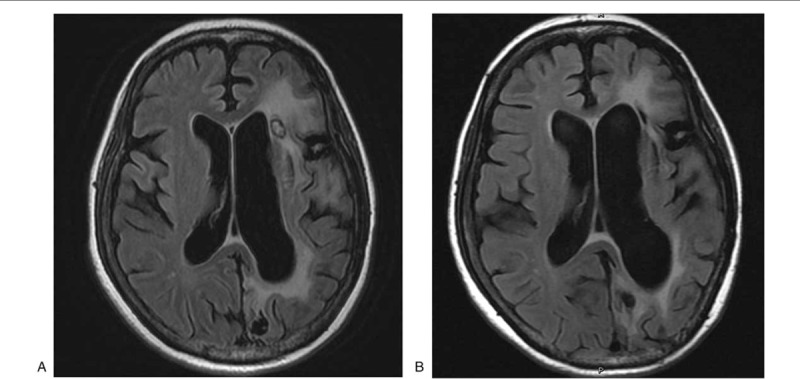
(A) MRI scan of a patient at the time of diagnosis of initial recurrence of glioblastoma. (B) Post-chemotherapy MRI scan of the same patient showing obvious shrinkage of tumor mass. MRI = magnetic resonance imaging.
